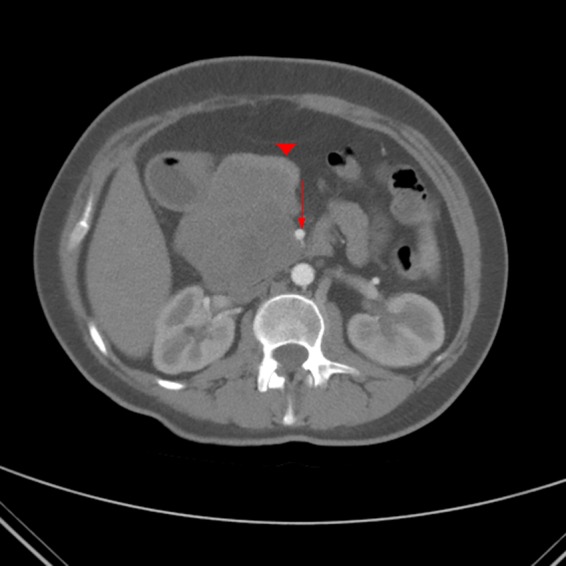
Preoperative contrast-enhanced abdominal computed tomography (CT) scan, axial arterial phase. The images reveal a low-density mass (*red arrow head*) measuring about 8.2 × 7.6 cm in diameter and prominently involving the uncinate process of the pancreas. The pancreatic head and neck are displaced and splayed around the anterior aspect of the tumor, certainly abutting the superior mesenteric artery (SMA; *red arrow*).
